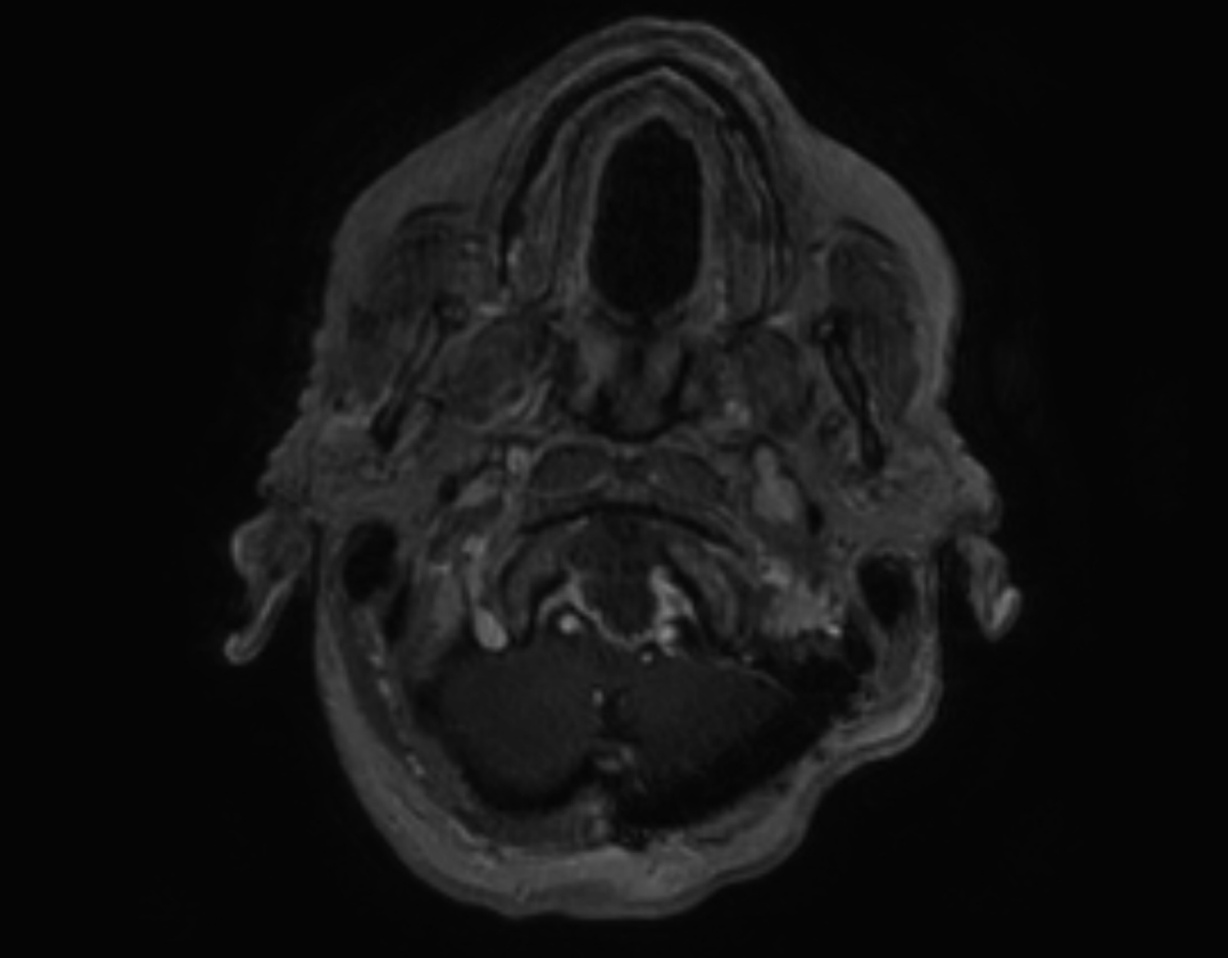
September Axial MRI Image 2 Foramen Magnum
